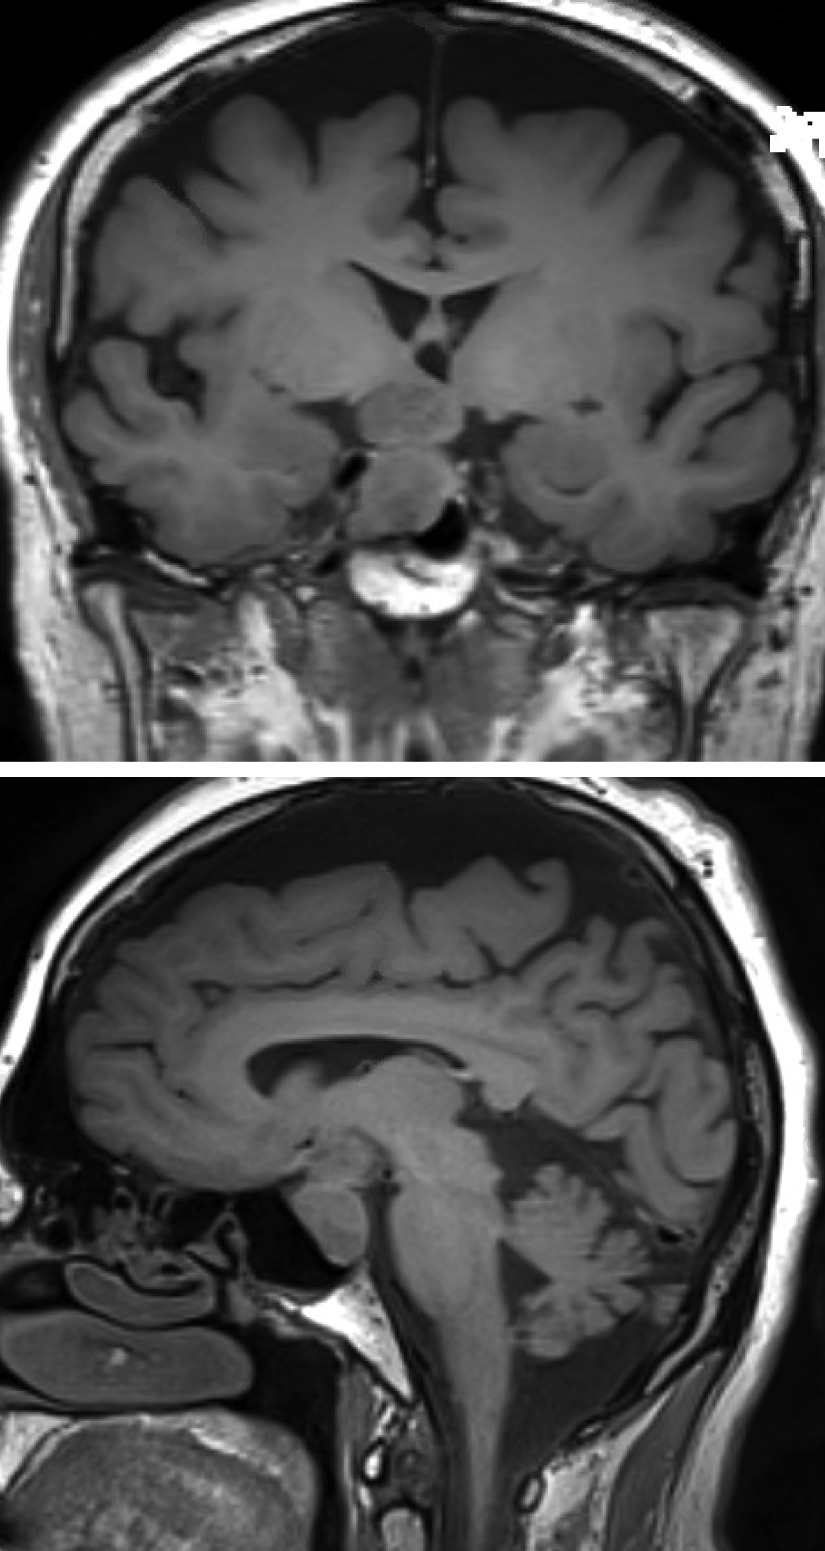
Postoperative pituitary MRI revealing residual isointense enhancement of the tumor in the sella and along the stalk measuring 9.9 × 9.9 mm in the anteroposterior (AP) and transverse dimensions and 1.3 cm in the craniocaudal (CC) dimension. The tumor is closely adherent to the posterior aspect of the optic chiasm
